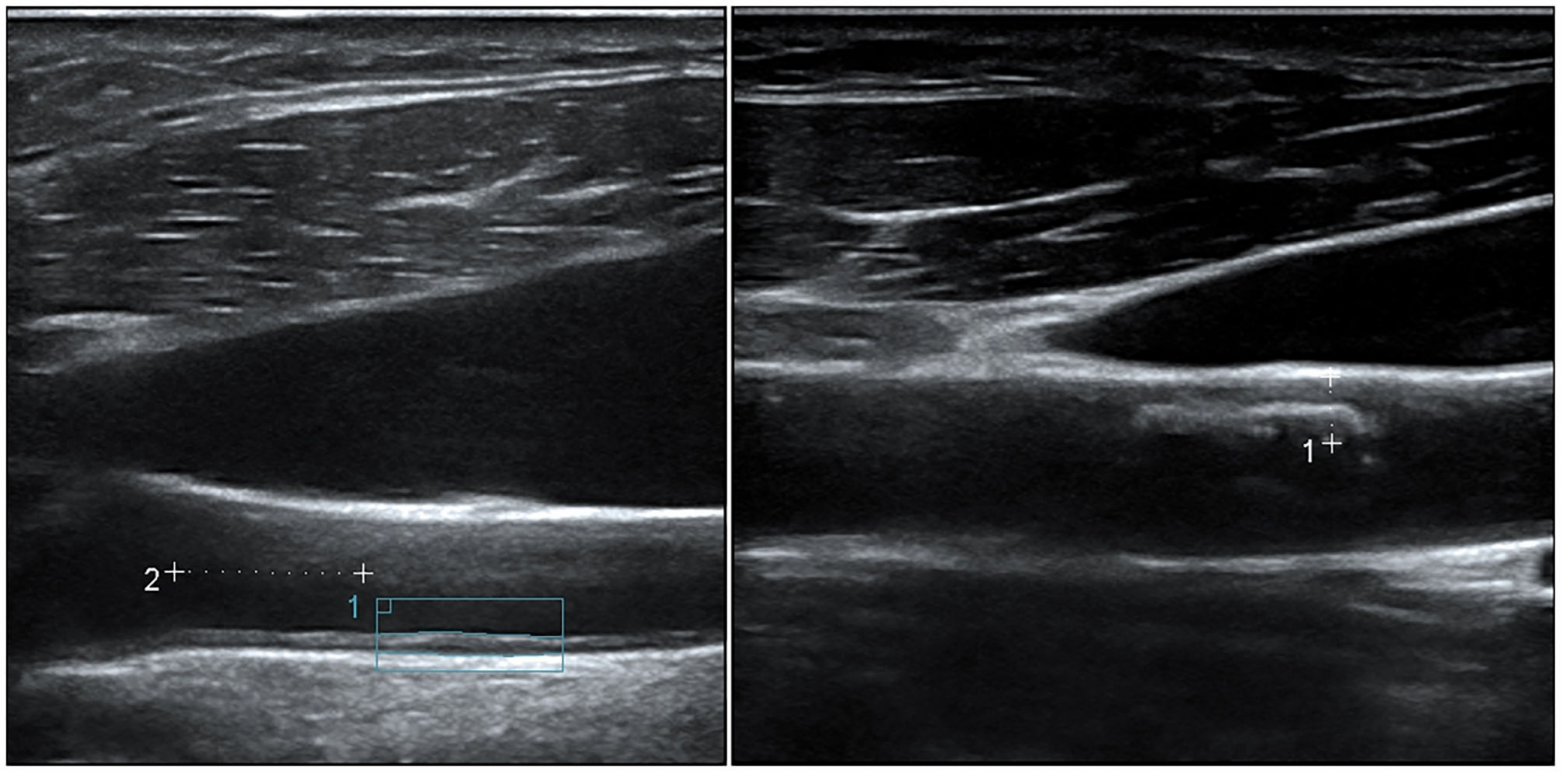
Representative ultrasound features of carotid atherosclerosis. (Left) An increased intima-media thickness (1.048 mm), measured 1 cm proximal to the carotid bulb according to the Mannheim consensus. (Right) A partially calcified atheroma plaque (maximal thickness 3.1 mm) at the anterior wall of the common carotid artery.
